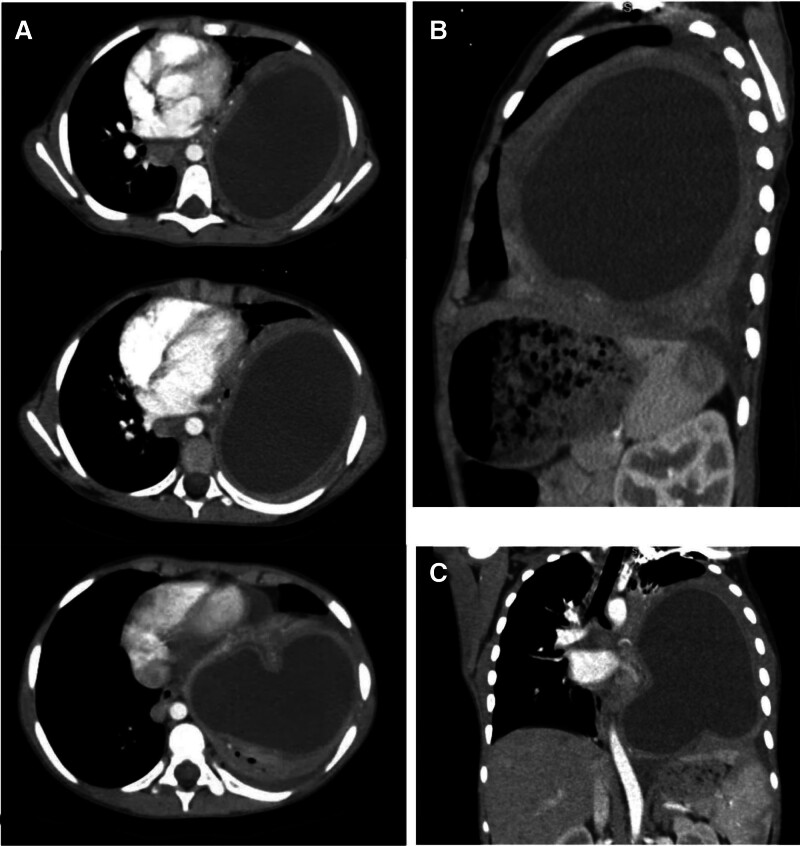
Chest CT scan with IV contrast (mediastinal window) shows a huge cystic lesion occupying most of the left hemithorax, with a marked mediastinal shift to the contralateral side. The cyst demonstrates fluid density, consistent with a large hydatid cyst or other cystic lesion. Significant compression of the surrounding structures, including the ipsilateral lung and diaphragm, is noted. (A) axial view, (B) sagital view, (C) coronal view.
